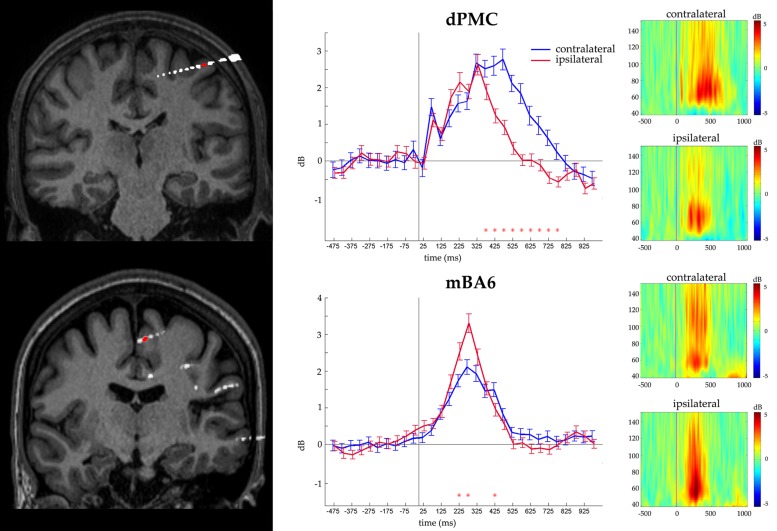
Representative recording from a dPMC (top) and a mBA6 (bottom) sites. (Left panel) The exact localization of the recording site is shown by the coregistration of the individual MRI with post- implantation CT. (Central panel) Gamma activity recorded from the contacts in red. Images show the statistical comparison between trials from the three condition pooled together, and requiring a contralateral vs. ipsilateral response in a -500, 1000 time window, in 30 adjacent time bins of 50 ms. Responses are aligned on the stimulus onset. Red asterisks indicate the significant *post hoc* comparisons. (Right panel) Time-frequency plots of the gamma activity (50–150 Hz) showing the frequency profile of the same electrodes, during contralateral and ipsilateral responses, in a -500, 1000 time window.
